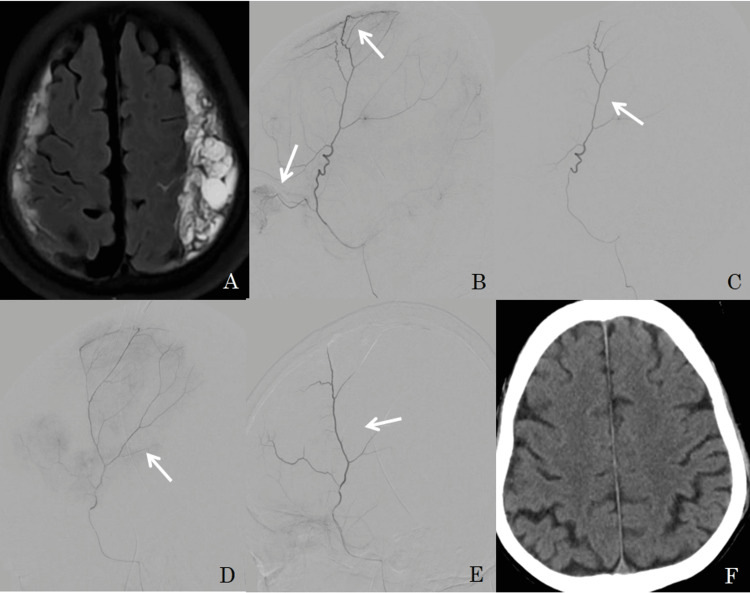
A 67-year female presented with bilateral SDH (image A), on selective catheterization of right MMA, shows tortuous frontal and parietal branches with abnormal blush (image B) and absent blush following embolization (image C) (arrow-head). Images D&E show pre and post-MMA embolization of left MMA, and complete resolution in a 6-month follow-up CT (image F).
